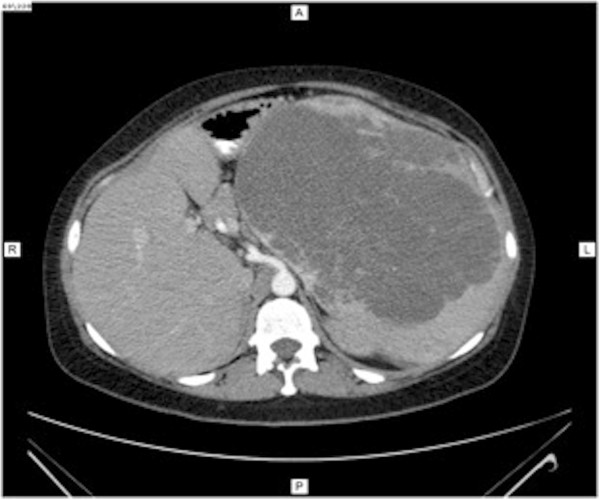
Computed tomography imaging of the splenic mass in arterial phase.
